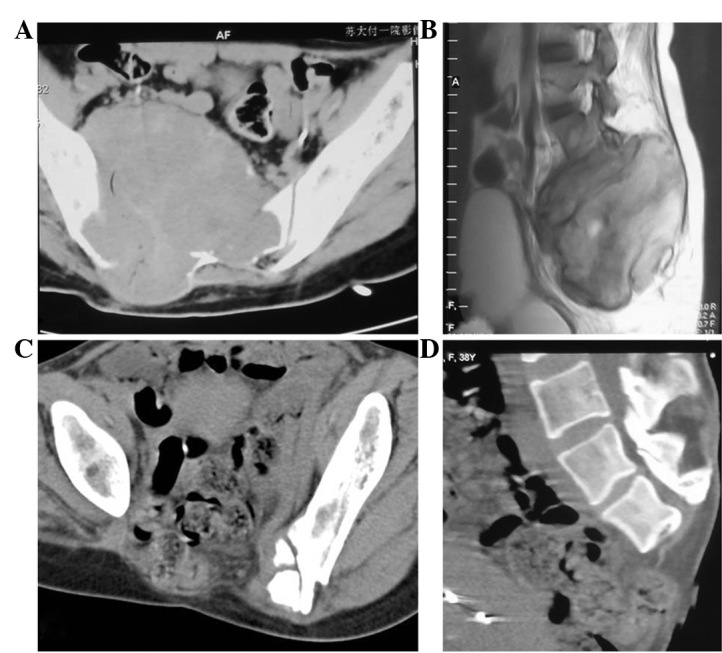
A 29-year-old female with a large giant cell tumor affecting the sacrum. (A) Axial CT scan showing an osteolytic lesion in the sacrum. (B) Sagittal T2-weighted MRI revealing a large soft tissue extension but no involvement of the surrounding tissue. (C) Axial and (D) sagittal CT scan of a 105 months post-surgical excision with pre-operative embolization showing no tumor recurrence. CT, computed tomography; MRI, magnetic resonance imaging.
